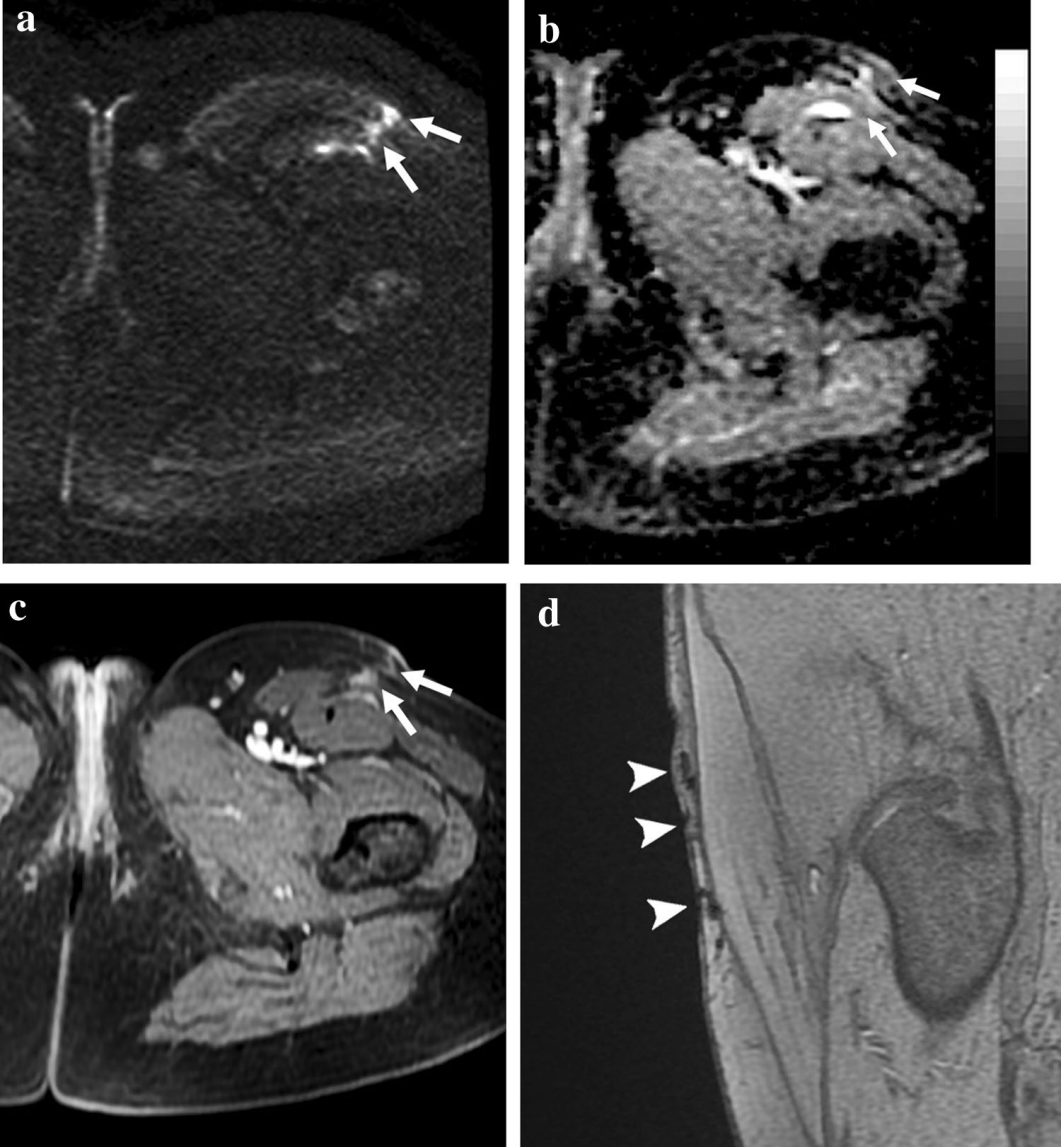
Effect of ferromagnetic artifacts on DWI. Forty-five-year-old woman MR follow-up after surgical excision of myxoid liposarcoma of the proximal left thigh. a Axial DWI showing tiny micronodular hyperintensities within the scar (*arrows*), corresponding to barely visible hypointensities on b ADC map. c Axial post-contrast fat-sat T1w GRE depicts a multinodular enhancement corresponding to DWI findings. d Sagittal T2*w GRE emphasizes the ferromagnetic nature of the artifacts (*arrowheads*)
